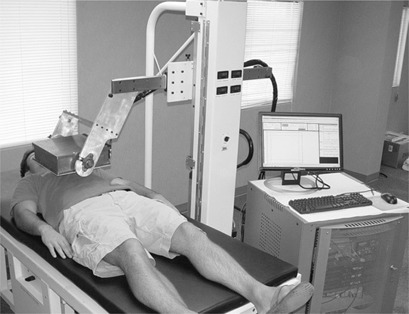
Mobile PET/SPECT system shown in a bedside position. Note the electronics rack to the right and a single detector positioned for SPECT imaging.
